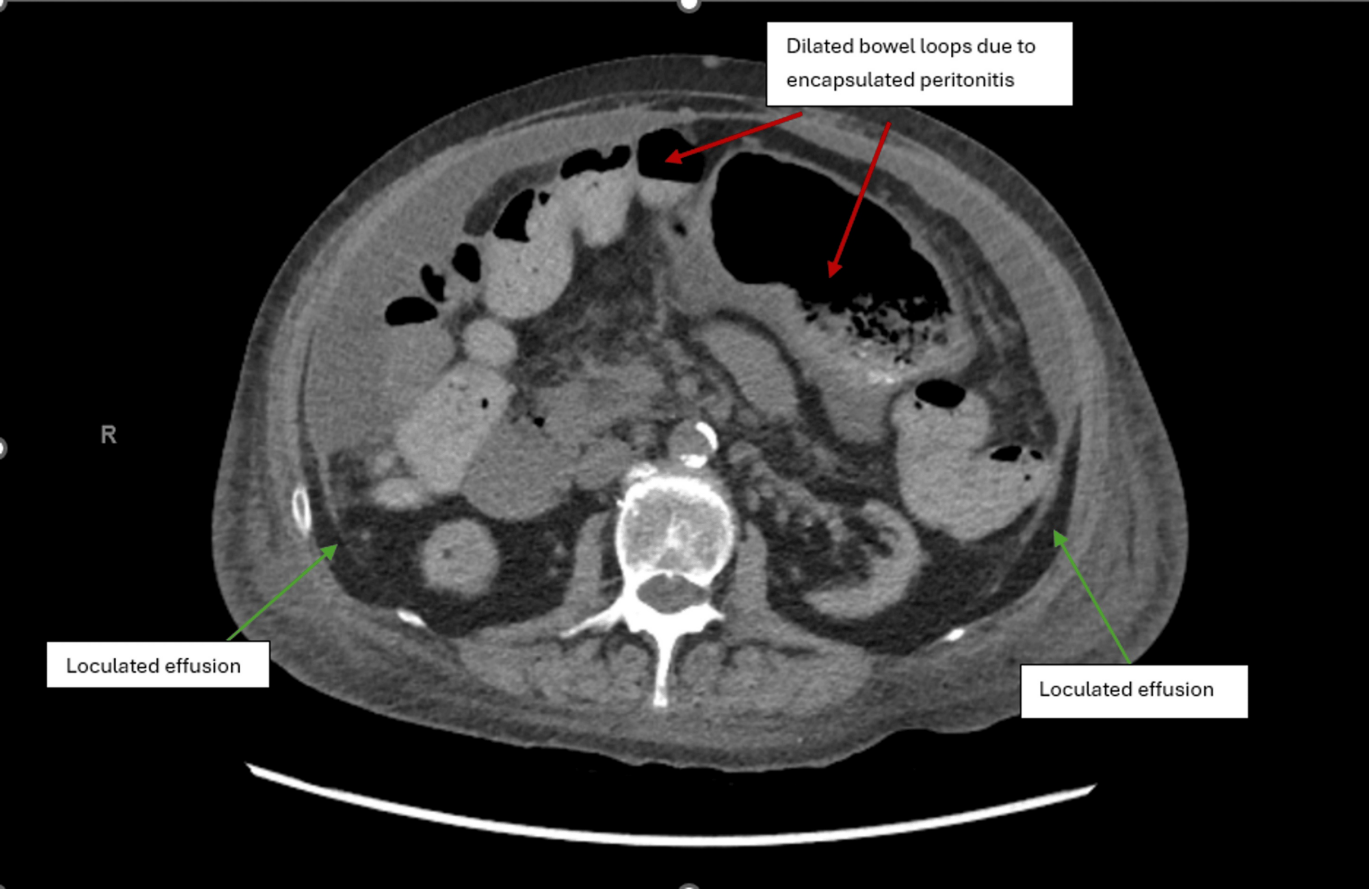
CT abdomen showing evidence of dilatation of the proximal small-bowel loops and loculated effusion resulting from thickening of the peritoneum
